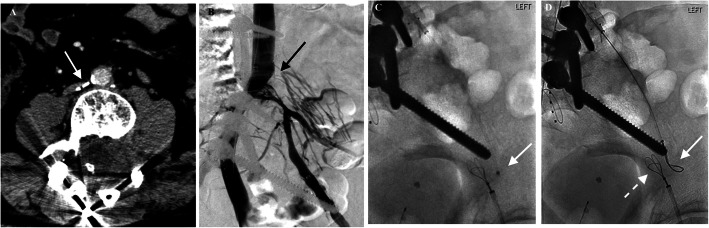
a CT demonstrated clips at level of LCIV origin (arrow) from prior surgical ligation of the LCIV, which were likely contributing to chronic occlusion of the vessel and complex recanalization techniques required; b Initial LLE venogram in the extreme RAO view shows flush occlusion of LCIV (arrow), in addition to postoperative findings related to L4 corpectomy and posterior lumbar fusion hardware; c Sharp recanalization needle was advanced from the proximal LCIV in the direction of the LEIV into the extra-vascular pelvic space (solid arrow), thus bypassing the known occlusion, confirmed on hybrid CT; d The two snares could now be advanced above (solid arrow) and below (dotted arrow) the area of occlusion and were confirmed to be close to each other on both AP and oblique projections
